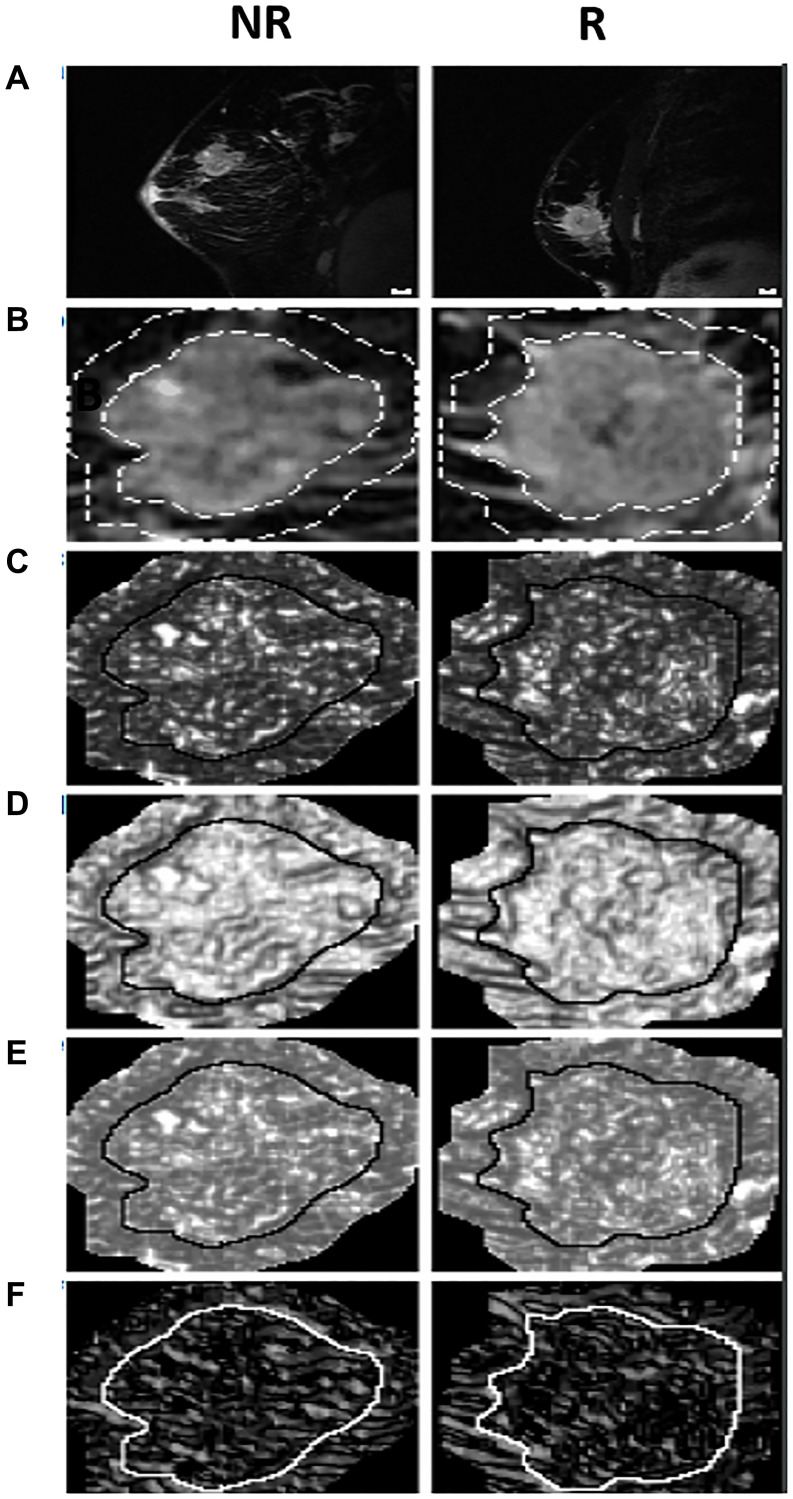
The MRI, quantized tumor ROI, and texture feature images for a typical NR and R. Includes (A) MRI sagittal view before treatment, (B) the MRI ROI quantized to 16 grey levels with both the ROI core and a margin of 10 pixels outlined, and feature images for (C) MAX, (D) HOM, (E) ENE, and (F) COR. The solid lines around the tumor core in the feature images differentiate the cores from the margins. Scale bars on the top panel correspond to 1 cm.
